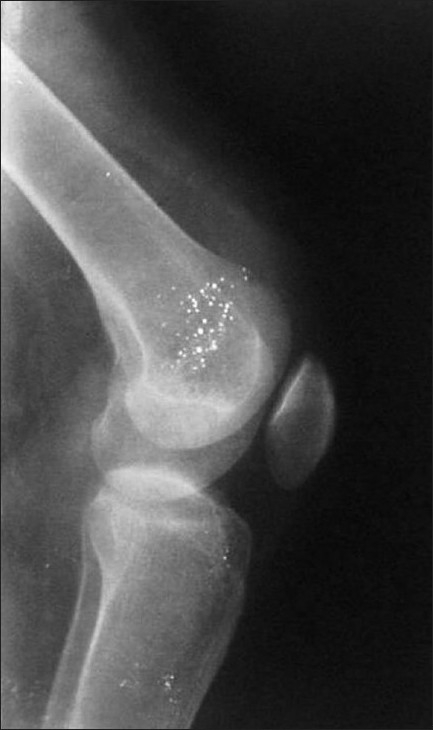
X-ray of lateral view of left knee shows inferior displacement of patella with loss of suprapatellar quadriceps bulk
